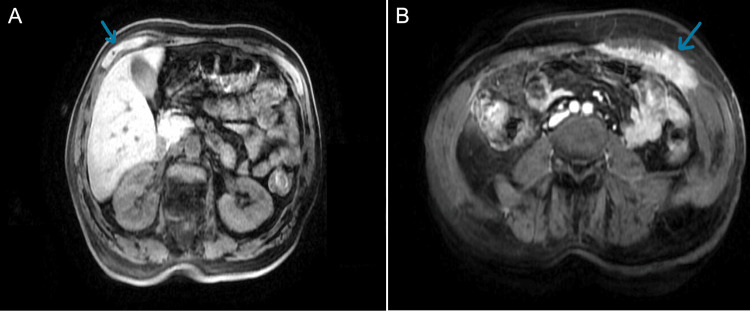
MRI scan after six cycles of chemotherapy showing left lesion in the muscles and a new lesion in the right rectus abdominis muscle (performed in 2019) MRI: magnetic resonance imaging
